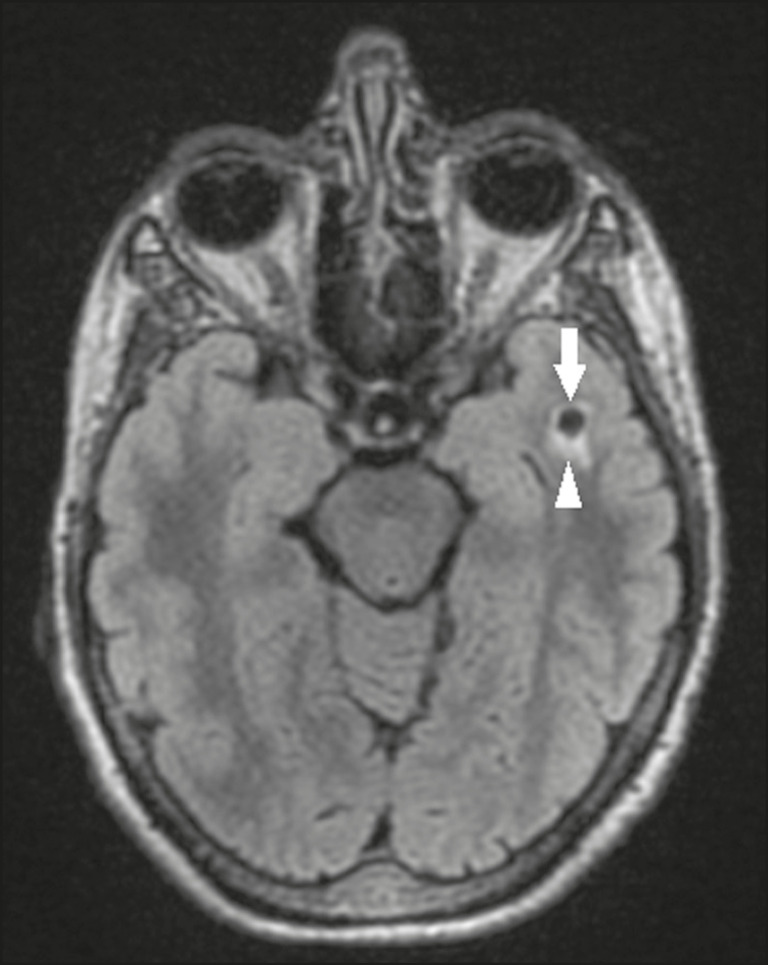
Enlarged perivascular space. Axial FLAIR MRI showing a small cyst-like lesion in the left temporal lobe with a signal similar to that of the CSF (arrow). Note the slight hyperintensity in the surrounding brain parenchyma (arrowhead).
